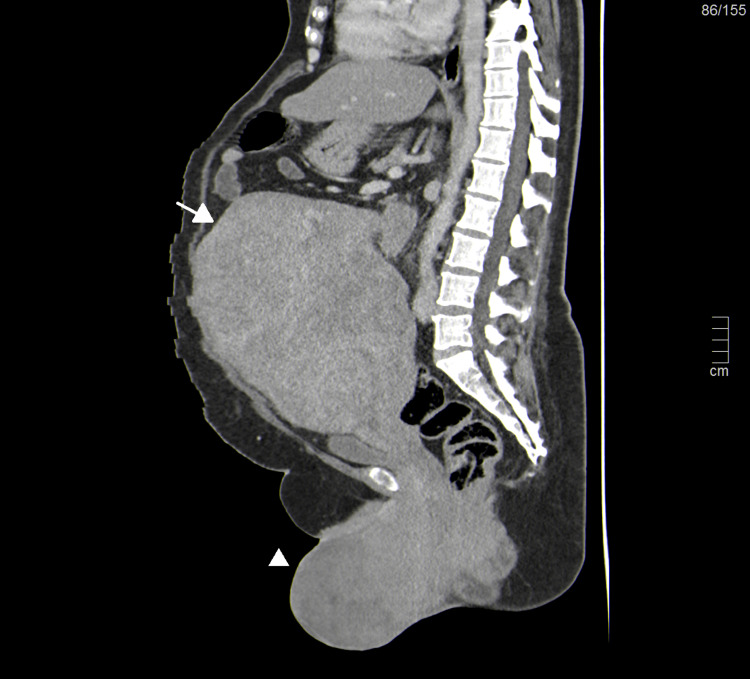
Computed tomography of the abdominal and pelvis saggital reformated plane showing enlarged abdominal (arrow) and pelvic masses (arrowhead) with regular contours and heterogeneous nodular enhancement
